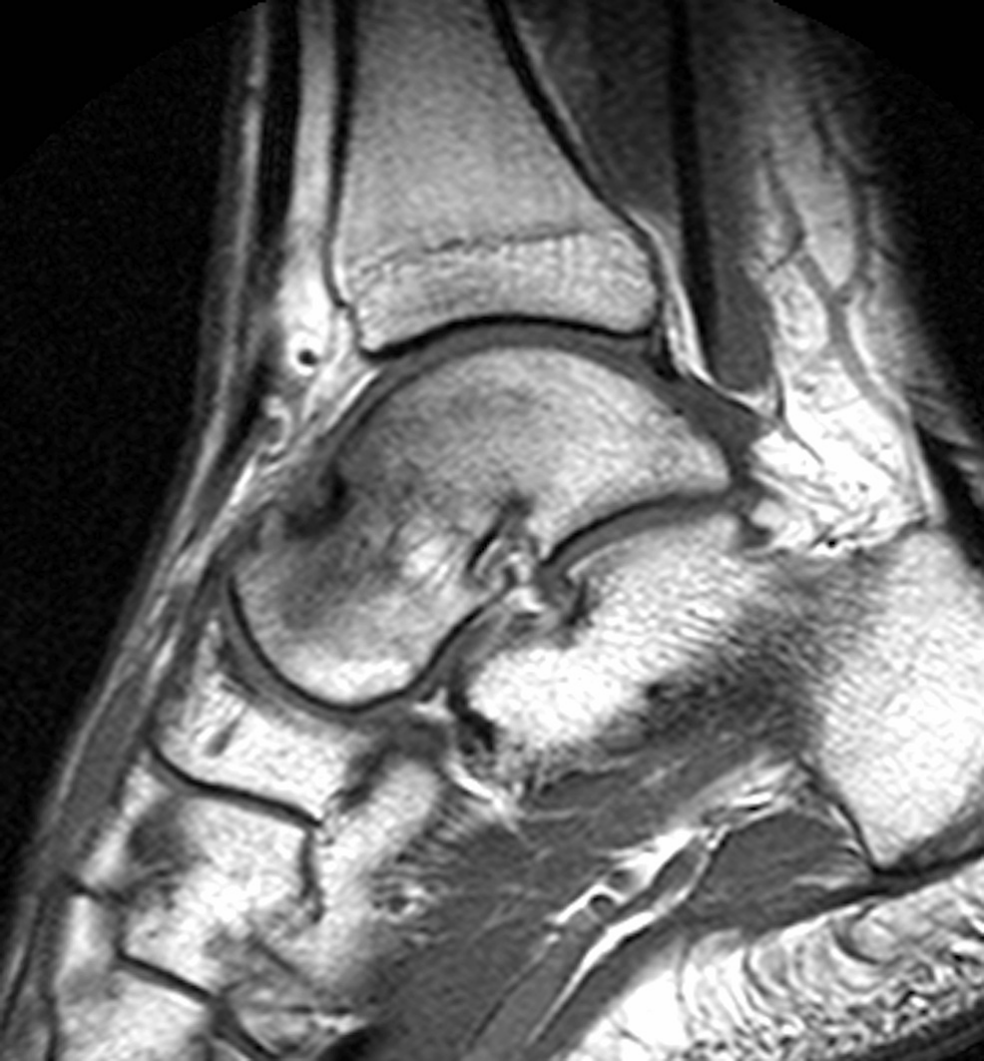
Magnetic resonance images indicated bone oedema and concomitant soft tissue inflammation at the talar neck.
